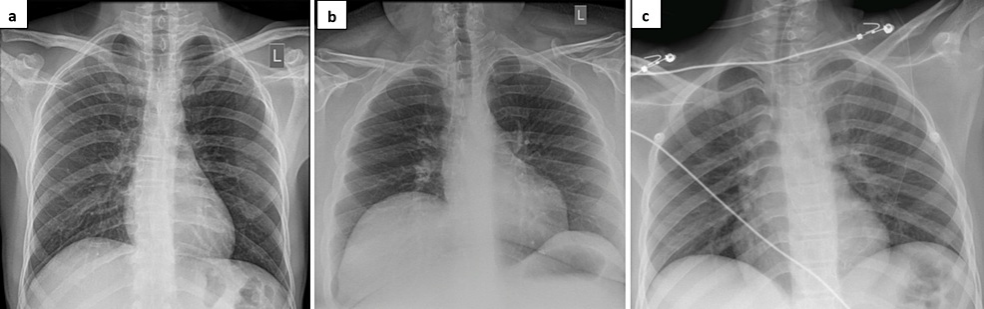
Chest X-ray images demonstrating the absence of features of COVID-19 pneumonia for case 1 (a), case 3 (b), and case 4 (c).
